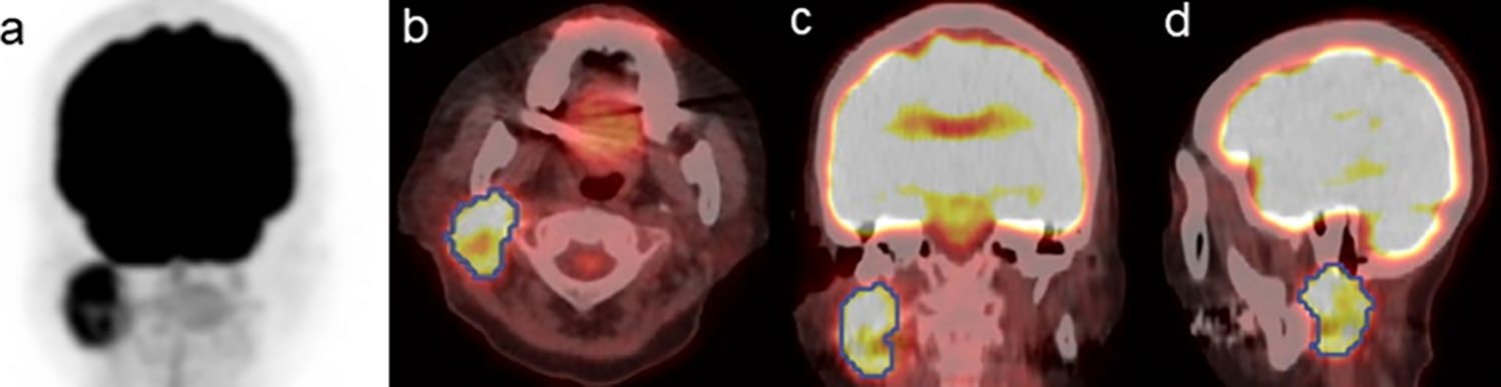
A 74-year-old female patient with a right malignant parotid gland tumor (squamous cell carcinoma). Pretreatment 2-deoxy-2-[^18^F]fluoro-d-glucose ([^18^F]-FDG) positron emission tomography/computed tomography scan images (maximum intensity projection [MIP] (a), transaxial (b), coronal (c), and sagittal (d)) revealed focal [^18^F]-FDG uptake in the primary lesion. The blue line represents the border of the volume of interest. The calculated probability score for predicting malignancy (positive ≥ 0.5) was 0.61 in the DL-based ensemble model. Thus, the DL-based ensemble model can predict the malignant parotid gland disease in this case
